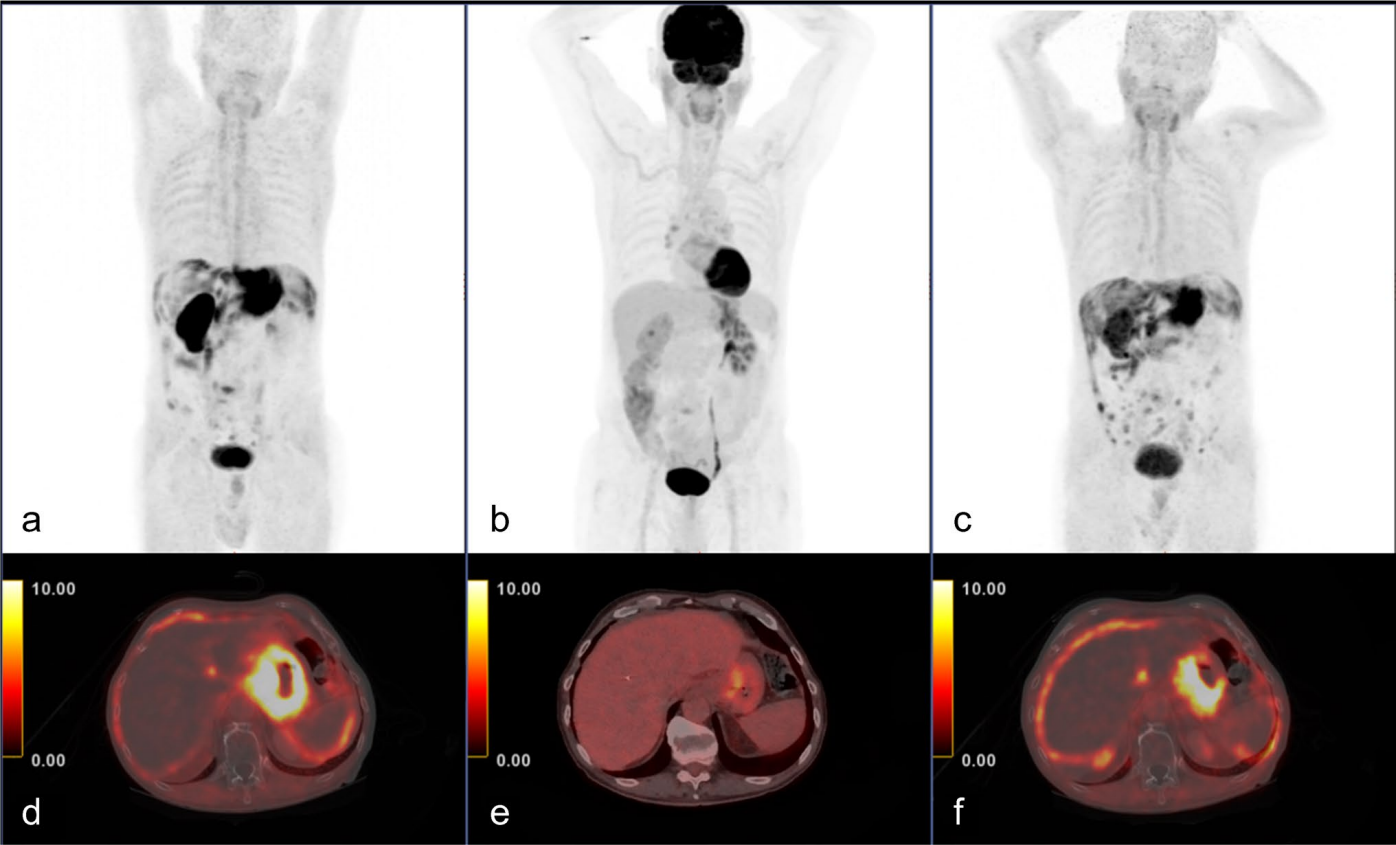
Staging FAPI (a, d) and FDG (b, e) PET/CT scans of a 78-year-old male with poorly differentiated gastric adenocarcinoma (patient #2 Table 1) showing intense FAPI uptake in the primary tumor and in peritoneal carcinomatosis compared with FDG (primary tumor SUV_max_ 23 and 6.8; primary tumor tumor-to-background ratio; 11.5 and 3.8; peritoneal-carcinomatosis SUV_max_ 7.5 and 2.3, respectively). The follow-up FAPI PET/CT (c, f) after 4 months of chemotherapy shows disease progression
